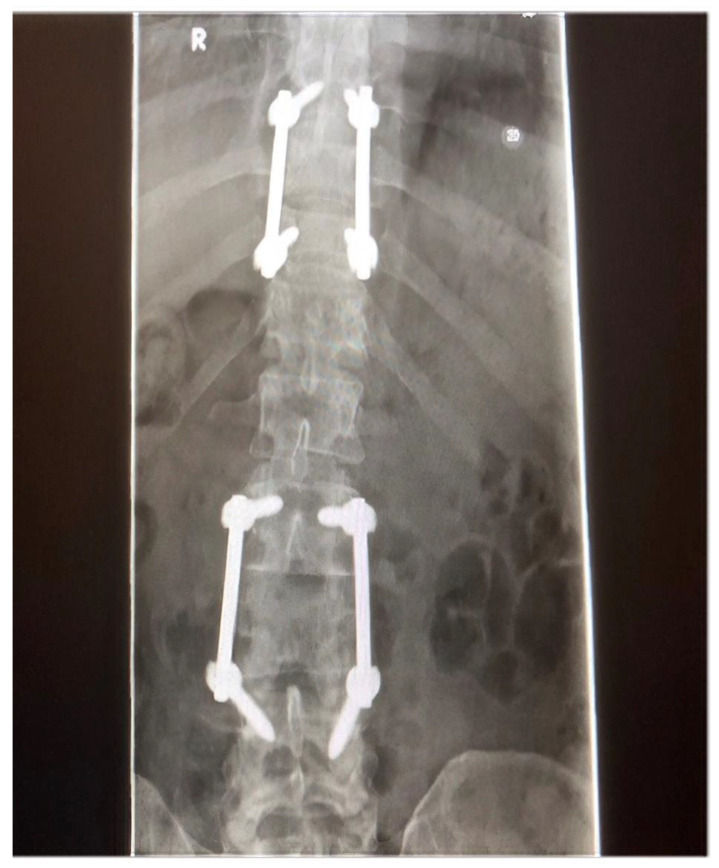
Post-operative x-ray demonstrating spinal stabilisation at the lumbar and thoracic areas using rods and screws.
